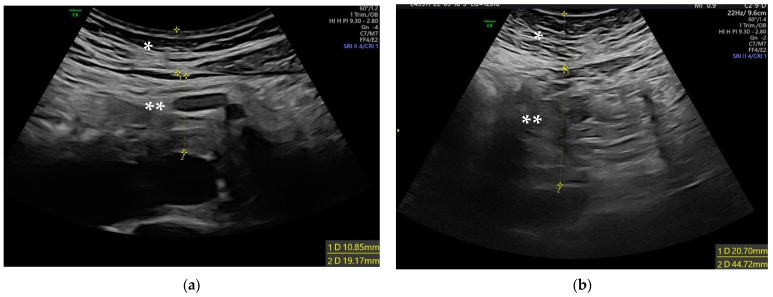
Representative abdominal first trimester ultrasound measurement of maximum subcutaneous fat thickness (*) and visceral fat thickness () in lean (a) and obese women (b).
